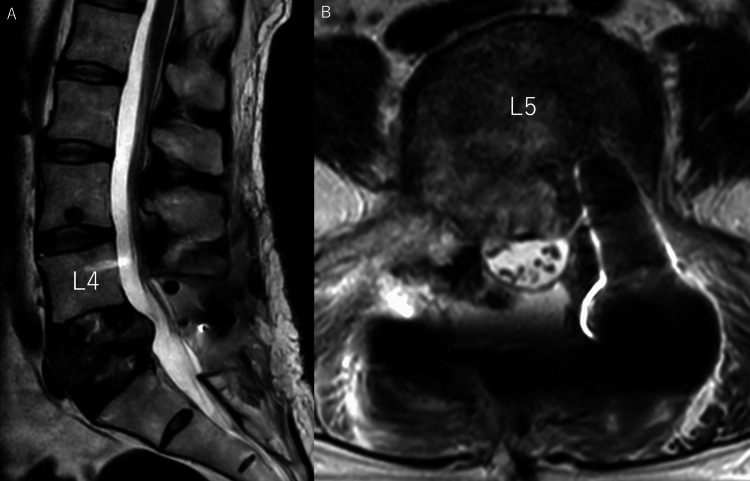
(A) T2-weighted MRI sagittal section, the first day after the surgery. (B) T2-weighted MRI transverse section, the first day after the surgery. A. The L5 vertebral canal stenosis at the L5 vertebral body level was removed by L5 laminectomy. A hematoma was seen posterior to the dural canal, but it did not compress the dural tube. B. A pedicle screw was found to have no signal area at the left L5 pedicle. The dural canal was well decompressed, though the dorsal tumor protrusion remained, while the L5 nerve root was not decompressed at the lateral side.
